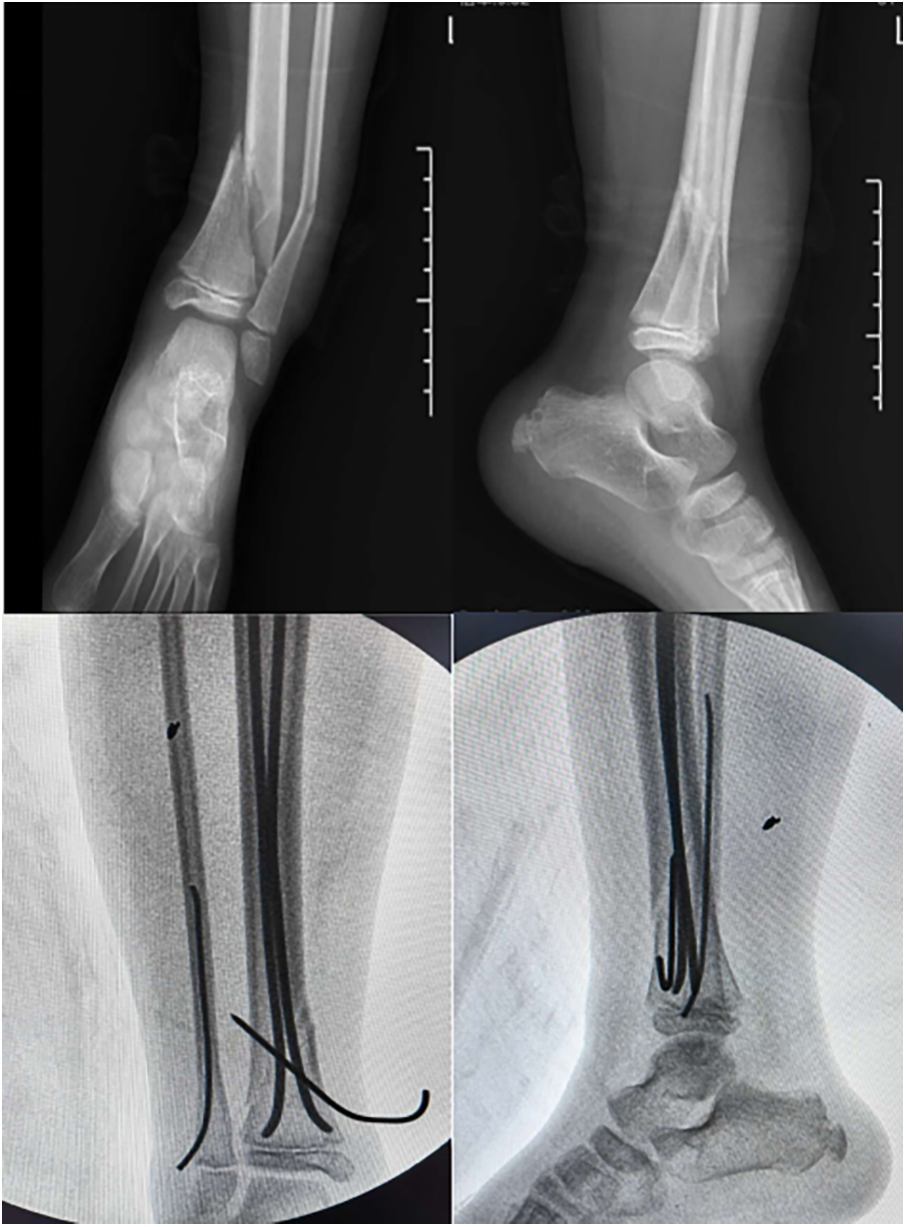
Case2: A 6-year-old girl presented with a fracture of the distal tibial diaphyseal metaphyseal junction(DTDMJ). X-ray films showed coronal varus deformity of the distal tibiofibular fracture with displacement. After treatment with E-K technique, the coronal varus Angle of the distal tibiofibular fracture was completely corrected.
